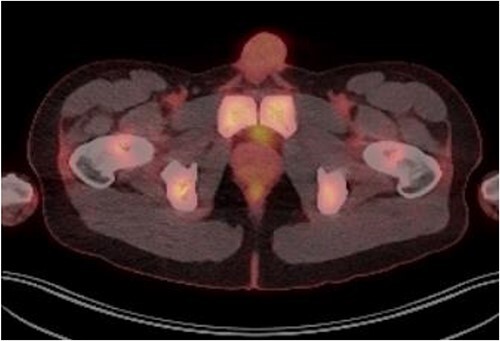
PET FDG scan post R-CHOP chemotherapy showing prostate with complete resolution from the disease.
